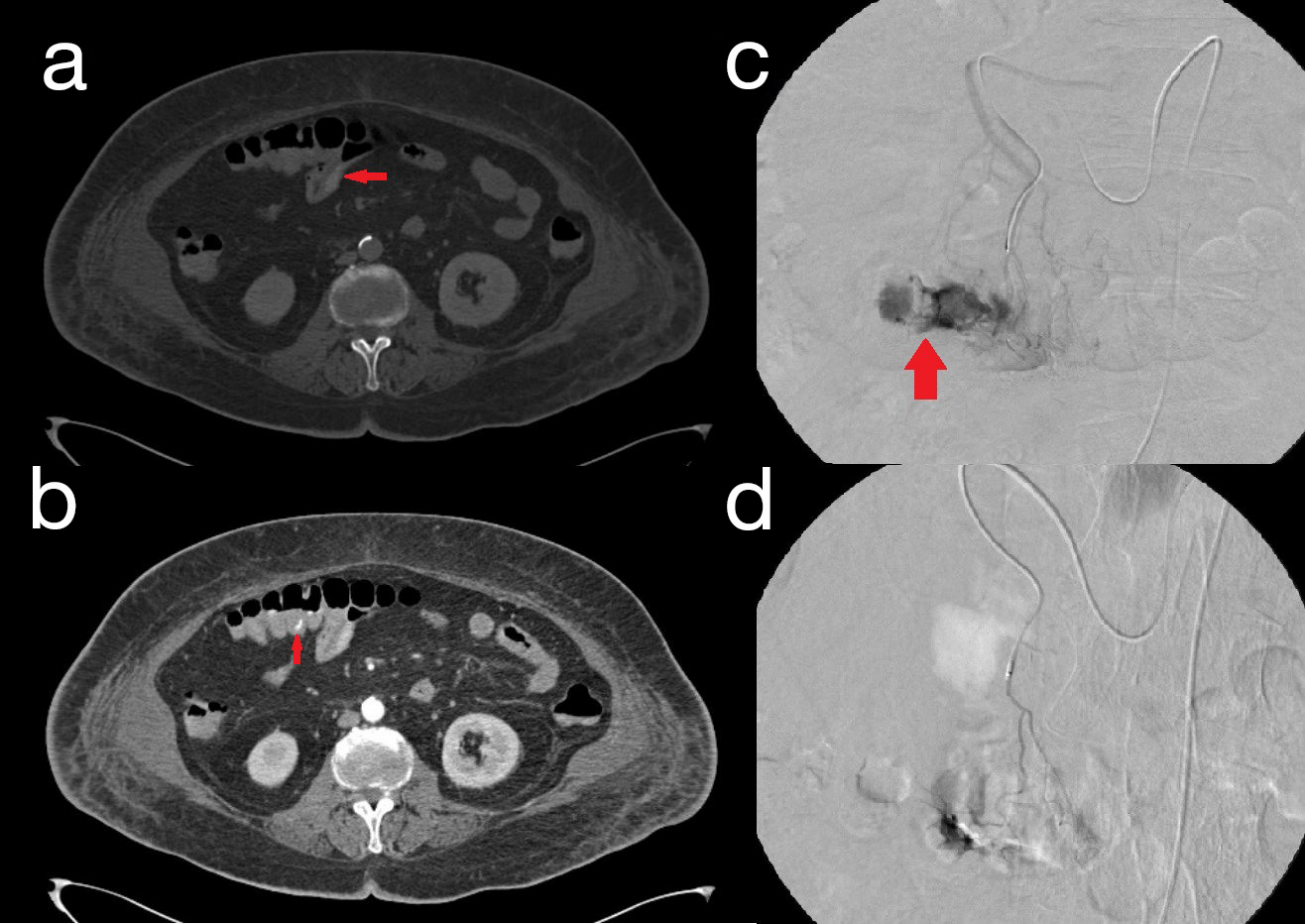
a 72 years old female presenting with rettorrhagy and anaemia. Pre (A) and post-contrast CT scans of the abdomen (B) show clots and active extravasation of contrast medium within transverse colic lumen, respectively (red arrows). DSA is thus performed: the presence of an active bleeding from a branch of the middle colic artery is confirmed (C; red arrow) and successful TAE with 2 ml Glubran 30% is performed (D).
